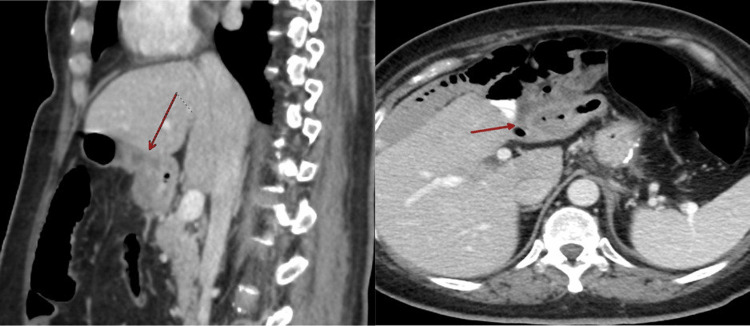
CT abdomen showing the gastrojejunal fistula (red arrow).
